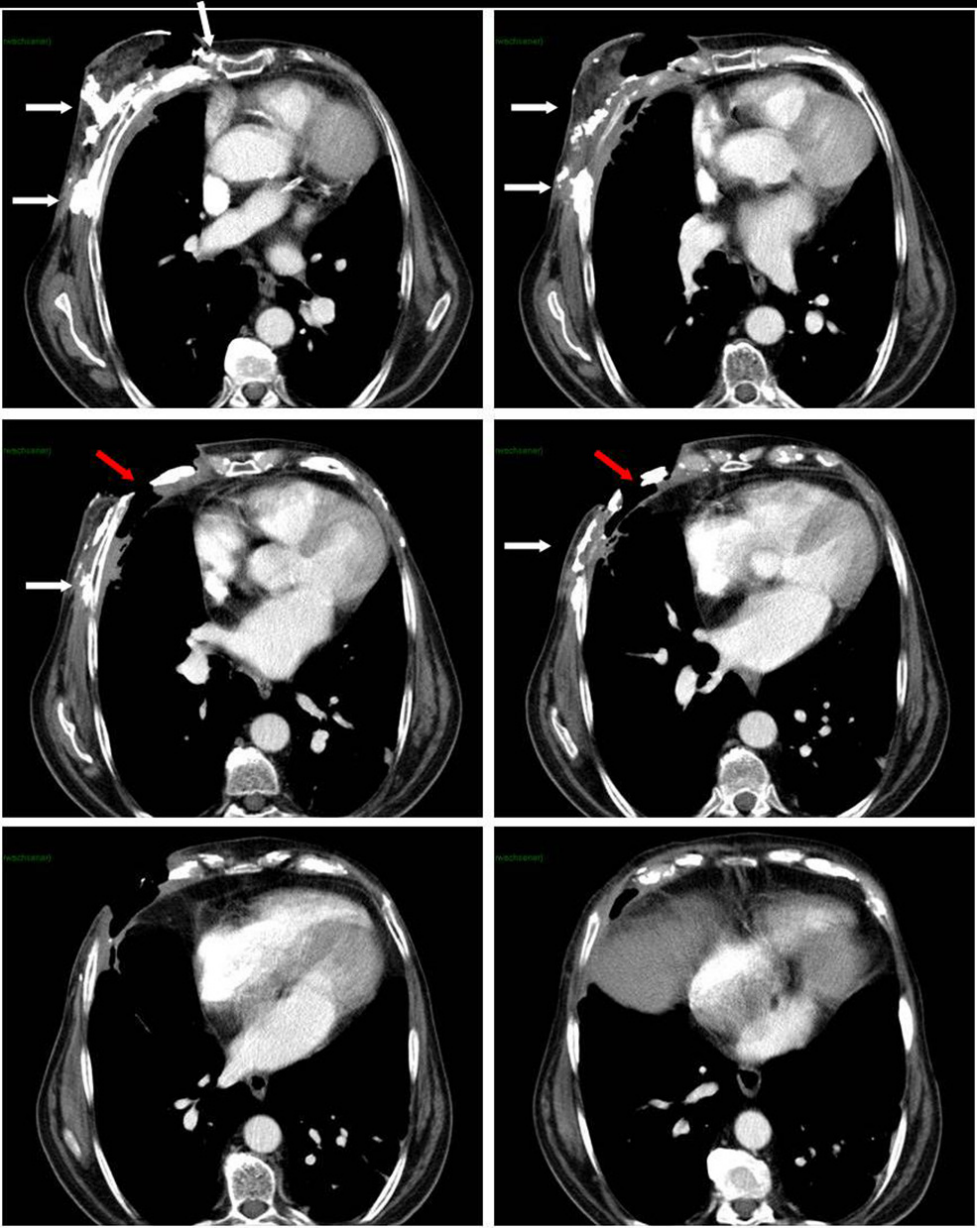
CT scan of the thorax after resection of necrotic and infected tissues: it shows a deep ulceration in the right breast extending to the pleura, remnants of a debruised fourth rib and heterotopic calcifications throughout the breast and thoracic wall tissues. White arrows indicate heterotopic ulcerations, red arrows a debruised rib.
